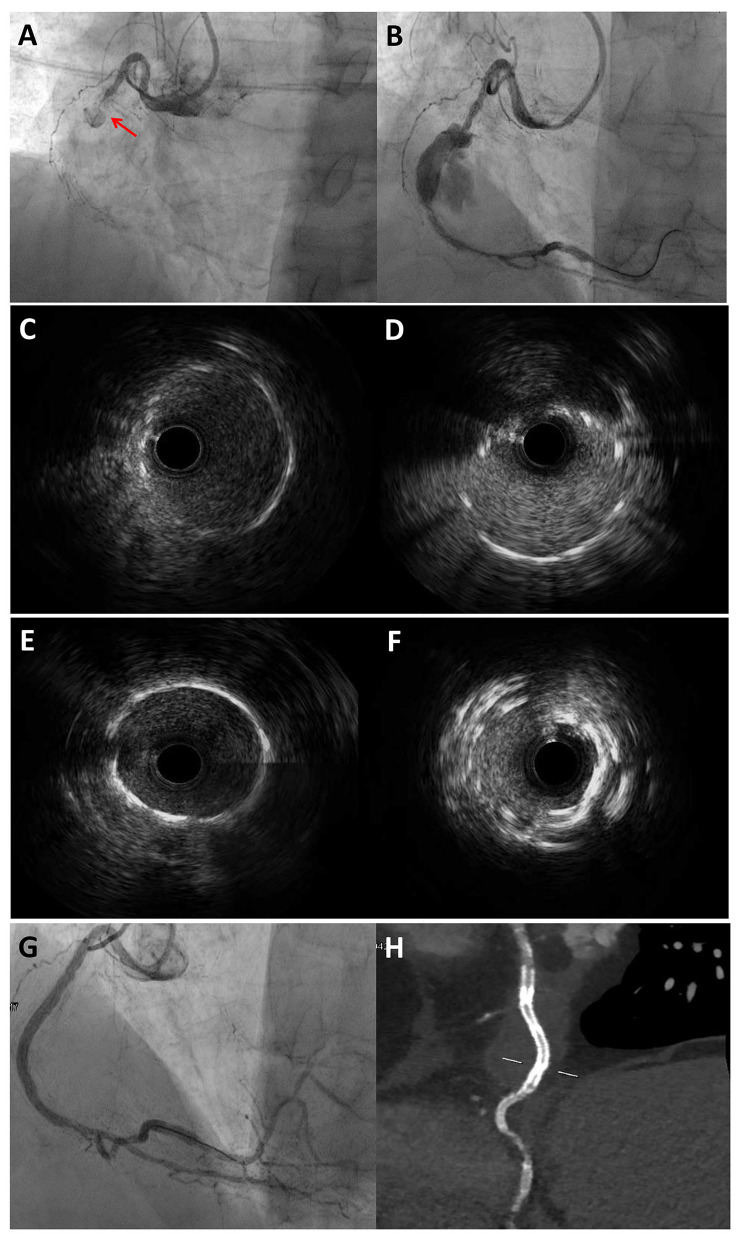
Multimodality imaging approach for diagnosis and PCI guidance in a STEMI patient with angiographic evidence of CAE. 77-year-old man admitted for STEMI, who underwent emergent coronary angiography. Coronary angiography showed an occlusion of the mid RCA due to stent thrombosis (A) and a CAA distal to the occlusion site, which was visible after guidewire crossing and thrombectomy (B). IVUS evaluation showed the previously implanted stents at the proximal (C) and distal (D) necks of the CAA. The PCI strategy consisted of 48 mm EES implantation bridging the proximal and distal CAA necks to create a supporting platform for the deployment of two overlapping covered stents. (E) and (F) show the proximal and distal edges of the EES assessed by IVUS. Coronary angiography showed an optimal sealing of the CAA after the implantation of two overlapping 3.5 × 24 mm single-layer PTFE covered stents (BeGraft, Bentley InnoMed, Hechingen, Germany) (G), which was confirmed by CCTA after the procedure (H). ^*^Courtesy of Dr. Iacopo Muraca. CAA, coronary artery aneurysm; CCTA, coronary computed tomography angiography; EES, everolimus-eluting stent; IVUS, intravascular ultrasound; PTFE, polytetrafluoroethylene; RCA, right coronary artery; STEMI, ST-segment elevation myocardial infarction.
